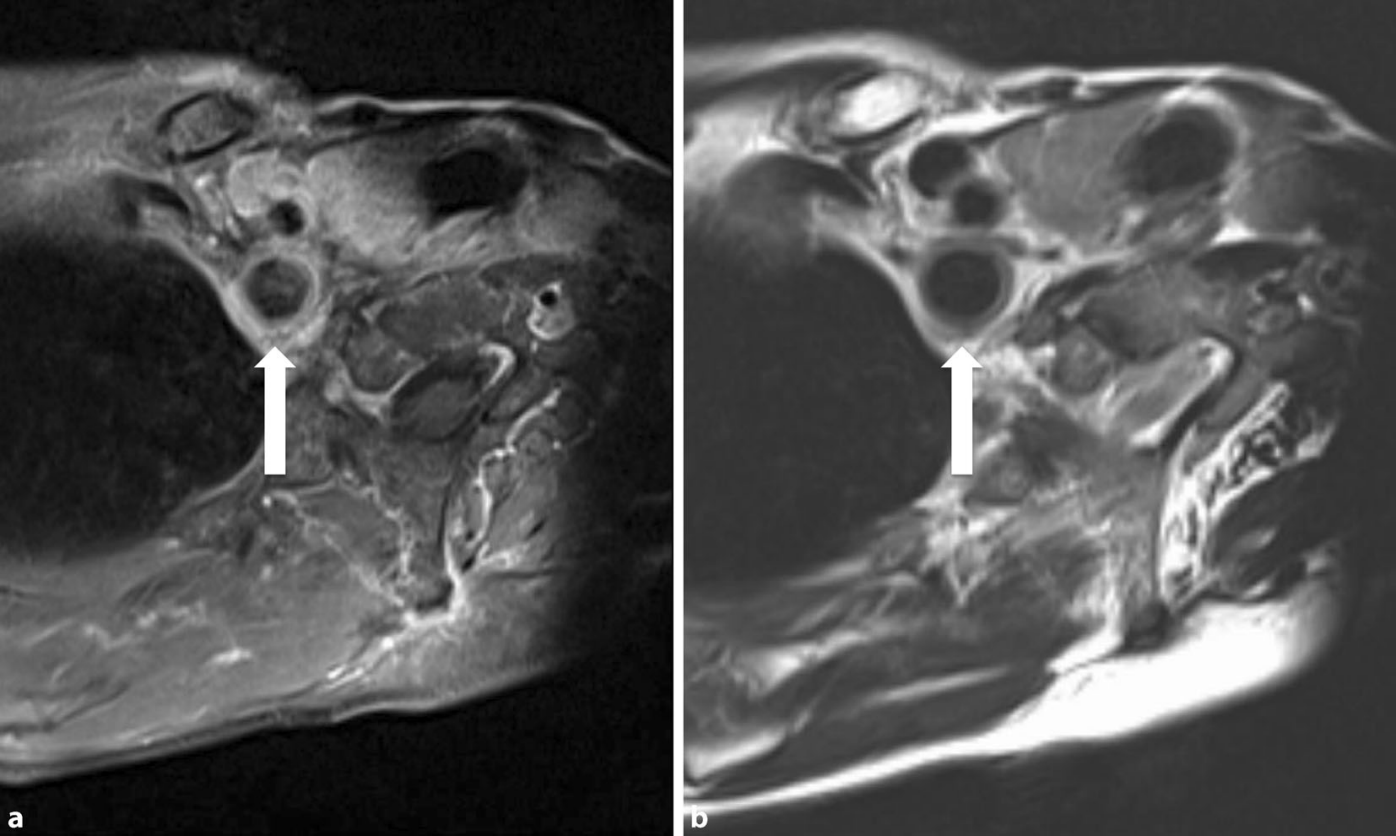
Magnetic resonance imaging showing (a) signal enhancement and (b) marked widening of the subclavian artery wall in a female patient with Takayasu arteritis (*arrows*)
